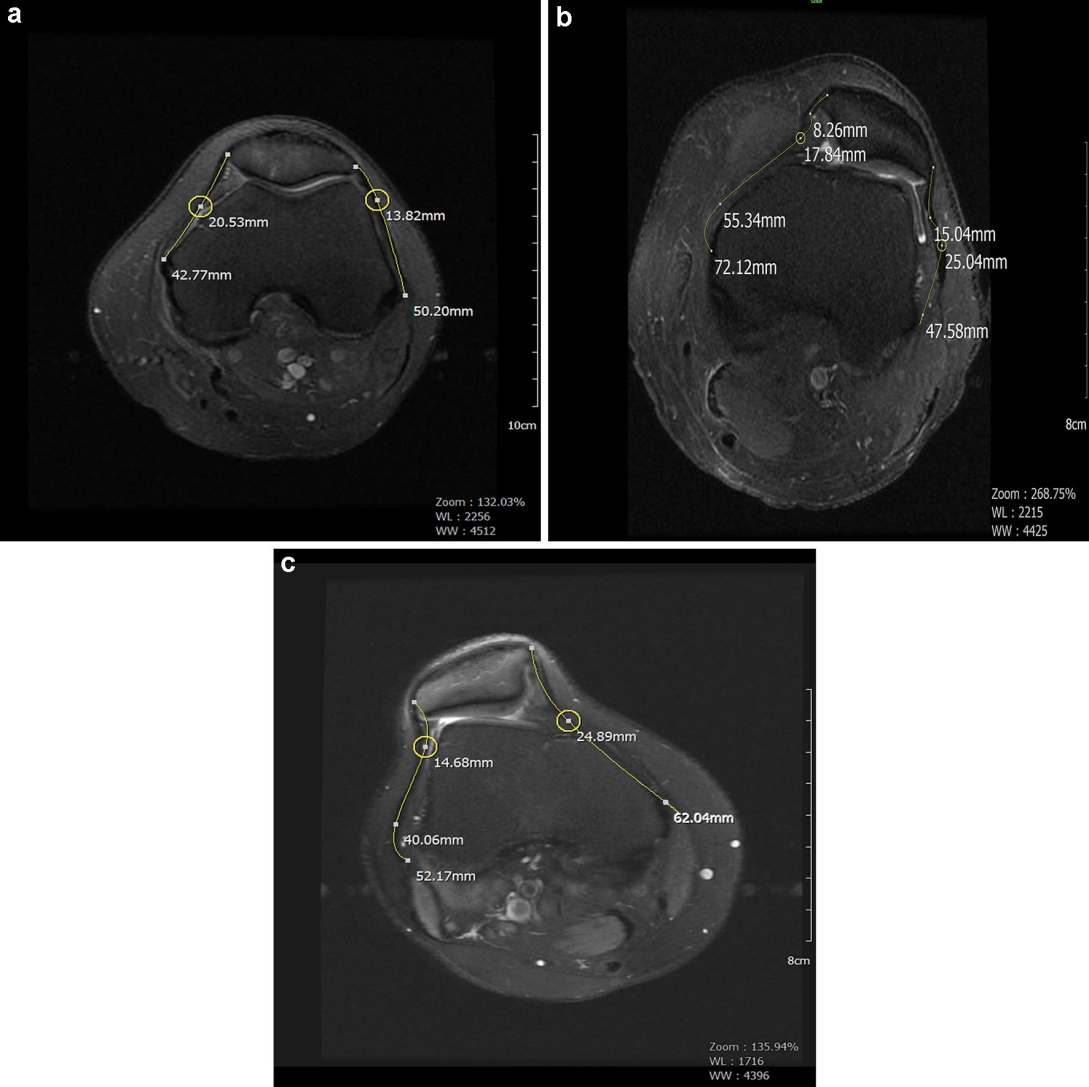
Medial patellofemoral ligament (MPFLL) and lateral patellar retinaculum length (LRR) measurements on axial PD-fatsat MRI crossing through the patellar center. a MPFL/LPR ratio of 42.77/50.20 = 0.85 was within normal limits. b This patient has type B dysplasia and patellar subluxation. MPFL/LPR ratio was 1.51, which was higher than the cutoff value. c This patient had type B dysplasia and patellar subluxation. MPRL/LPR ratio was 1.19
